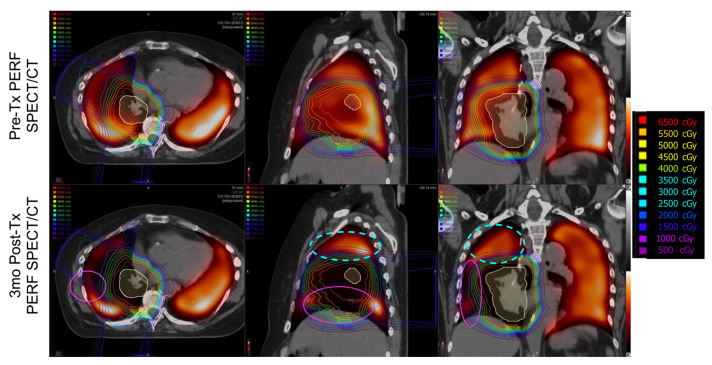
Taken from Thomas et al. [26]. Observed radiation dose–response on longitudinal perfusion SPECT/CT. (Upper row) Pre-treatment lung perfusion SPECT co-registered to planning CT and radiation isodose line rainbow overlay. (Lower row) Three-month post-treatment perfusion SPECT co-registered to planning CT and radiation isodose lines (rainbow overlay). SPECT window/level were normalized to out-of-field integral uptake. Regions within the treatment field show reductions in uptake that are correlated with radiation dose magnitude and spatial distribution. A dotted cyan oval is drawn to indicate a region that received low dose but did not experience perfusion decline. A magenta oval is drawn in another region that received low dose but did experience perfusion decline post-RT and is fed by an irradiated region. Reprinted with permission.
